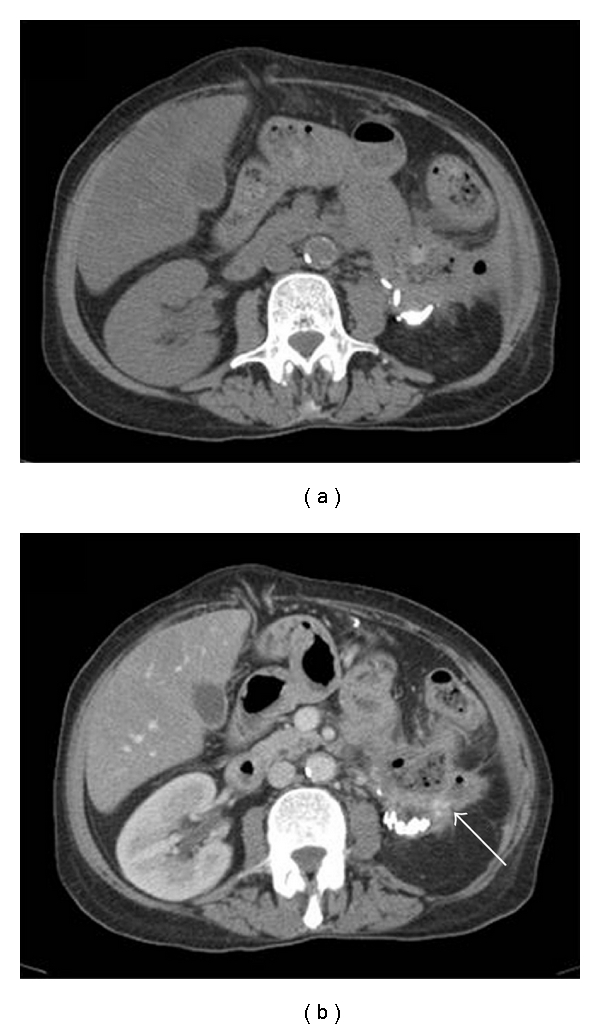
CT scan of the abdomen and pelvis. (a) Imaging without i.v. contrast. Stranding and thickening of the soft tissue immediately below the lower pole of the left kidney and around the splenic flexure. (b) Imaging after i.v. contrast application. Stranding and thickening of the soft tissue around the kidney appears more prominent (arrow).
